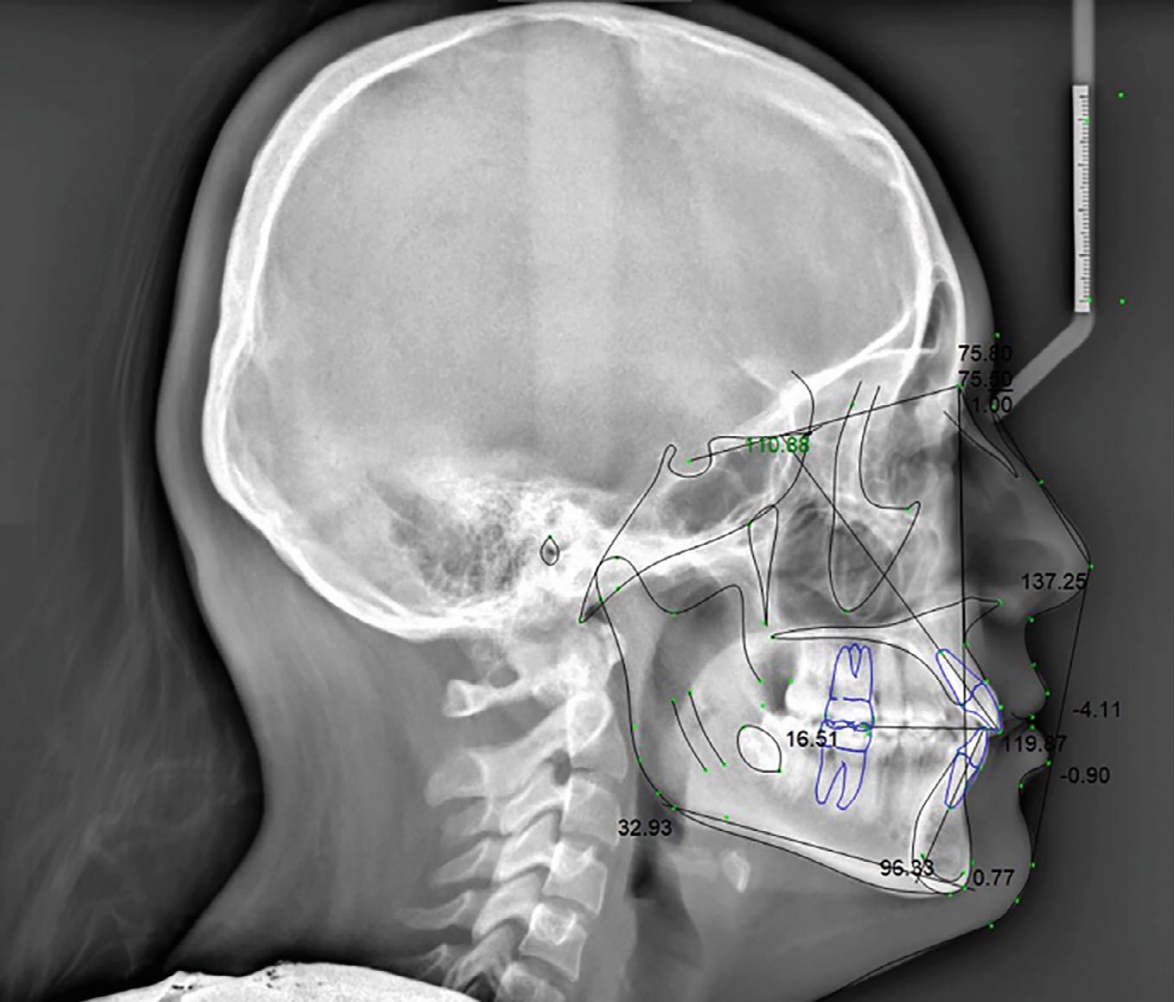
Posttreatment digitized lateral cephalometric radiograph.
